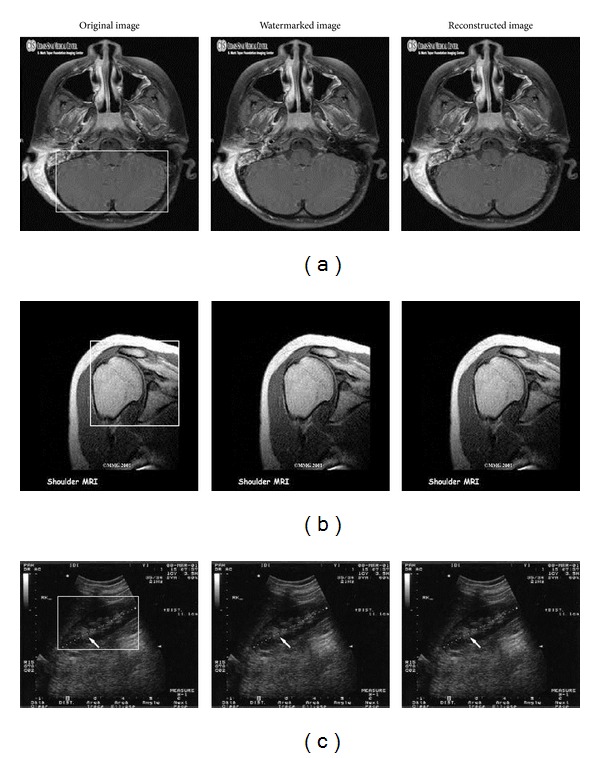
Original, watermarked, and reconstructed medical images. From top to bottom: CT scan, MRI scan, and ultrasound images.
